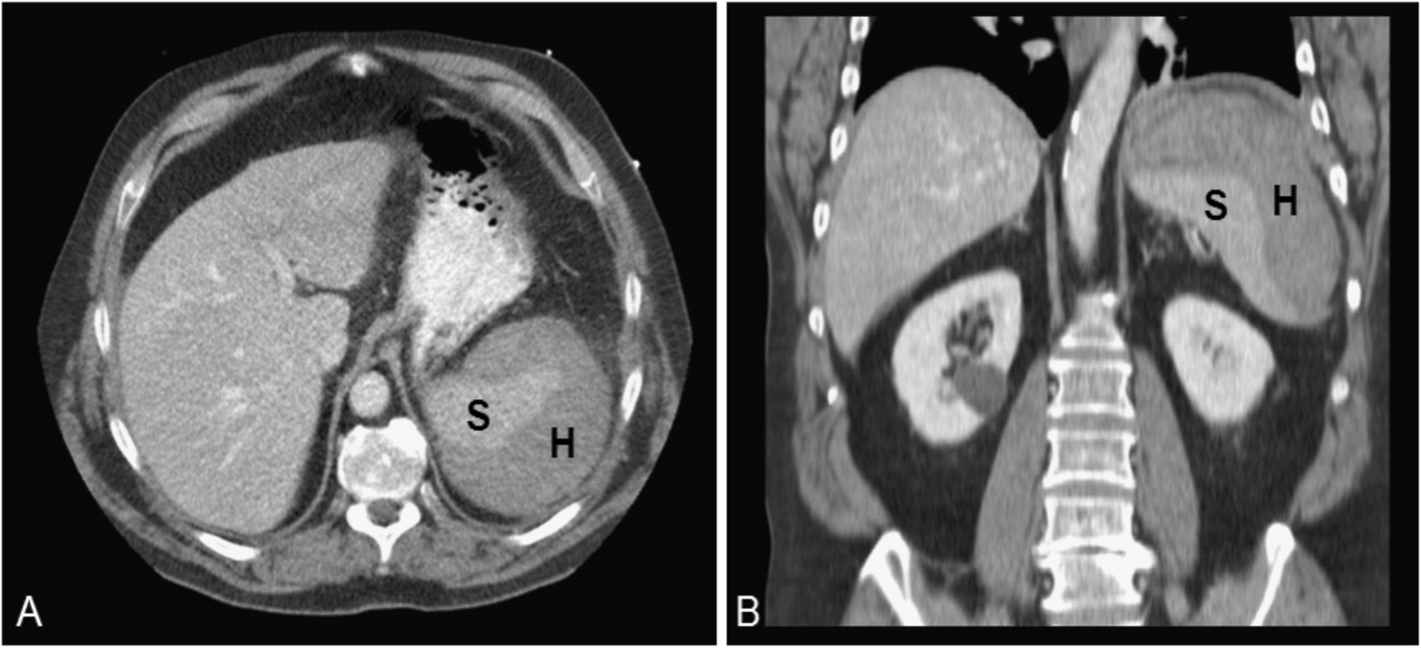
Contrast-enhanced computed tomography image with 5-mm slides in transverse (A) and coronal (B) orientation. Spleen (S) with large subcapsular hematoma (H) with an estimated volume of 0.75 l.
